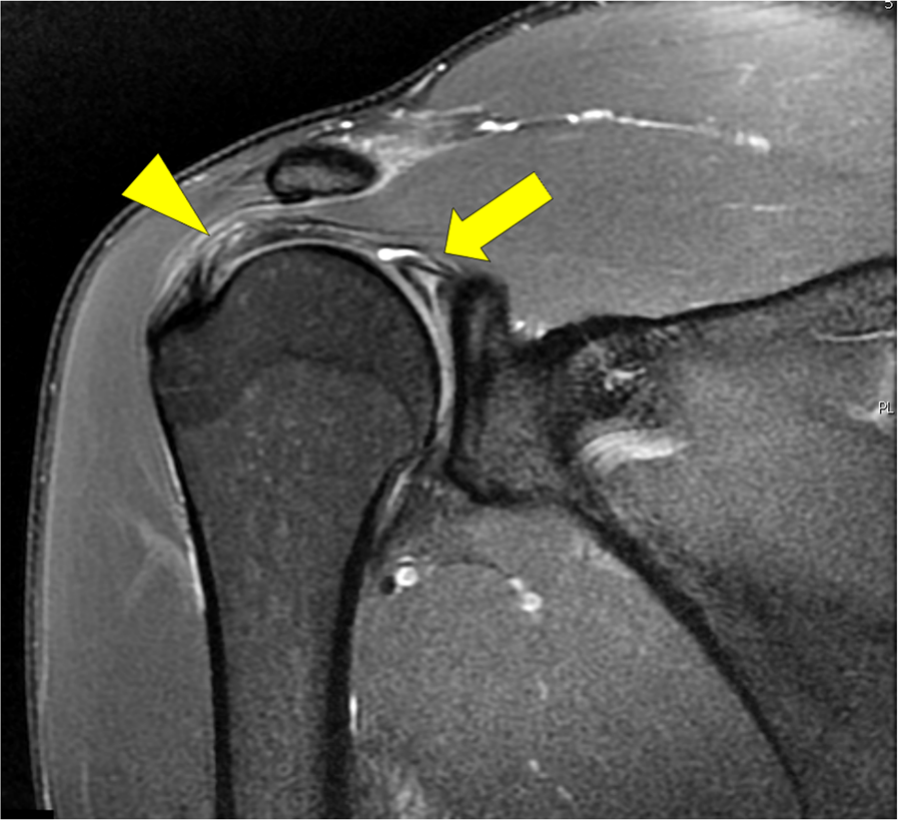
Male gymnast in his late 20’s: Coronal T2-weighted fat-suppressed MRI shows superior labral tear and overlying paralabral cyst (arrow) and a low grade intrasubstance tear of supraspinatus tendon (arrowhead)
